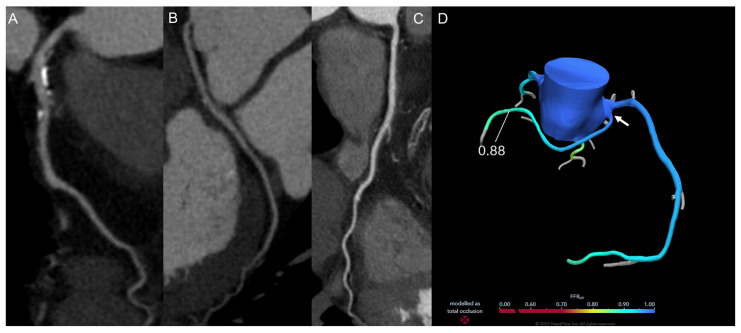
A 75-year-old woman, a former smoker, who had dyslipidemia, hypertension, and mild carotid artery disease, presented with effort dyspnea and atypical chest pain. CCT showed mild left main and left anterior descending artery disease (A) as well as an anomalous origin from the right coronary sinus and retro-aortic course of the left circumflex artery (B). The right coronary artery was free from disease (C). FFR-CT assessment was within normal range, including in the left circumflex artery (arrow; (D)). The patient was medically managed with no adverse events at follow-up.
